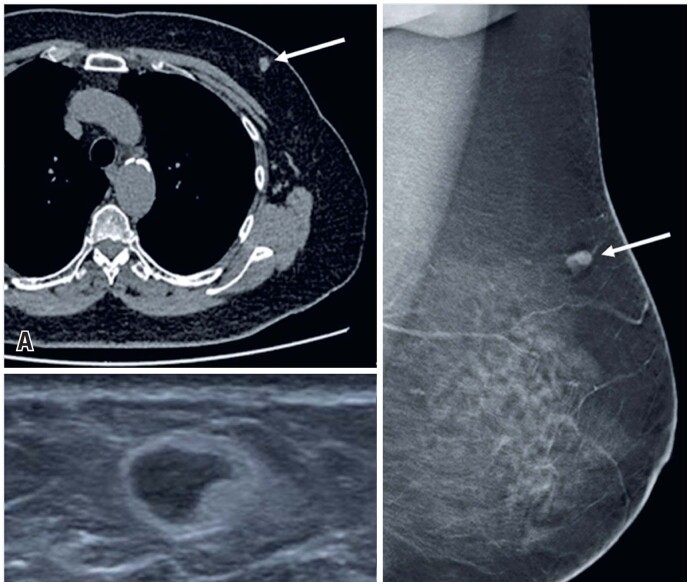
A 68-year-old woman underwent a chest CT for colon tumor staging. (A) The study identified a mass in the union of the upper quadrants of the left breast (arrow). (B) A breast ultrasound revealed an oval-shaped nodule. (C) Mammography showed a nodule suggestive of an atypical intramammary lymph node (arrow). The anatomopathological result was adipose tissue with fibroblastic reaction to hematologic material (*hematoma in organization*)
